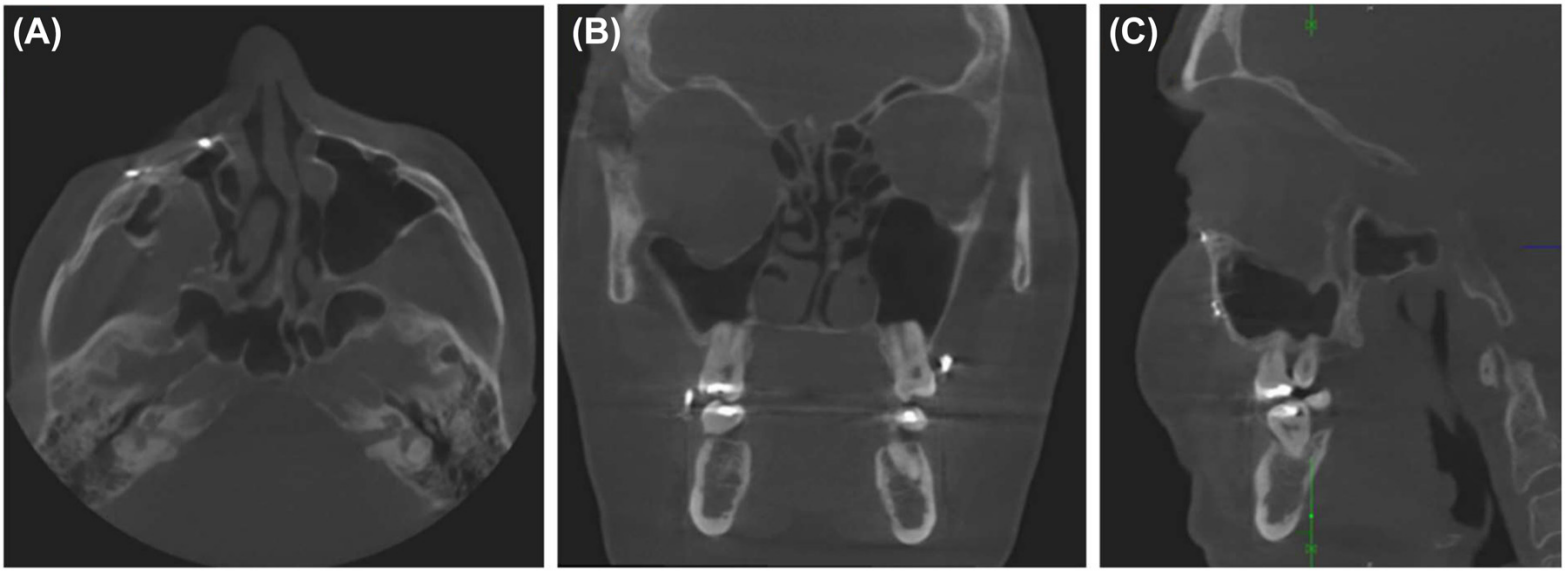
Cone beam computed tomography prior to secondary reconstruction of the patient in Figure 1. The deformity is apparent along all axes: The axial view (A) shows dislocation of the zygoma. The coronal (B) and median-sagittal (C) views show an increase in the orbital volume due to dislocation of the orbital floor.
